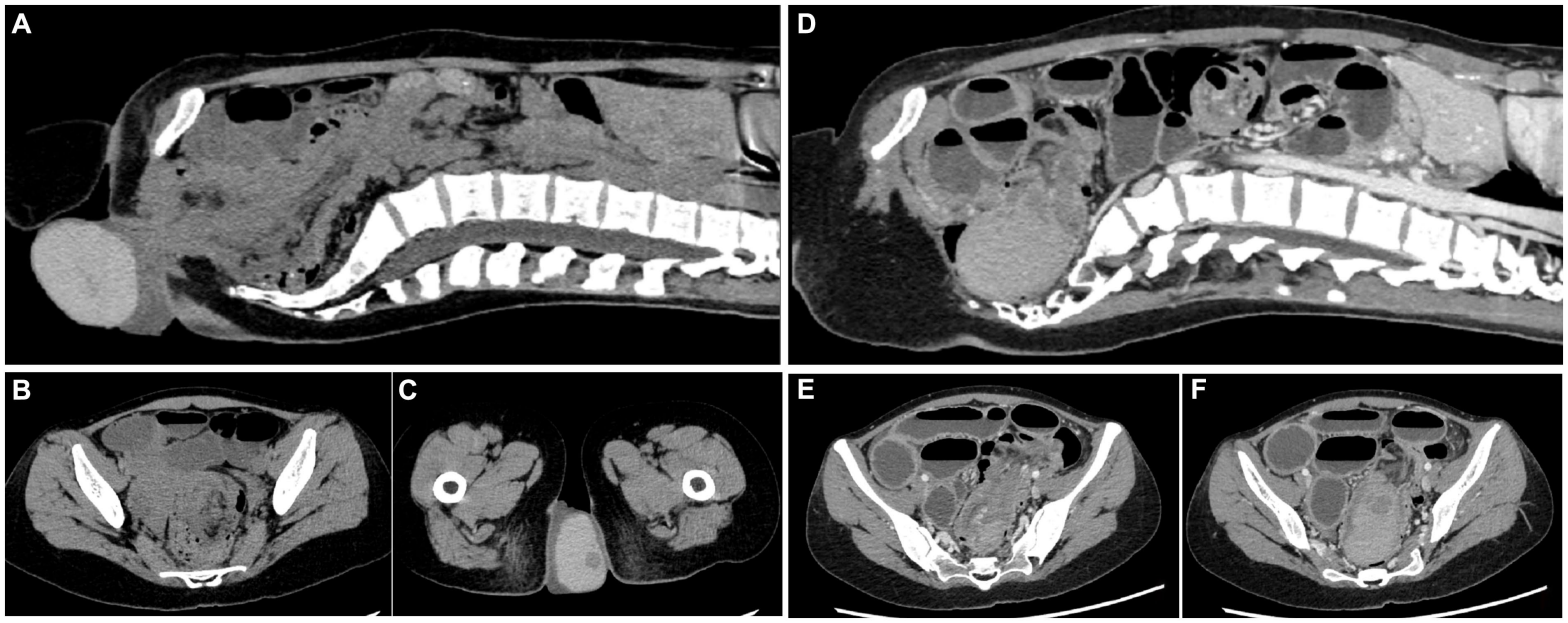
Computed tomography of the abdomen and pelvis. (A–C) Plain CT before the return of the cyst. (D–F) Enhanced CT after the return of the cyst. The intestinal duct structure is disordered, and the small intestine is obviously dilated.
